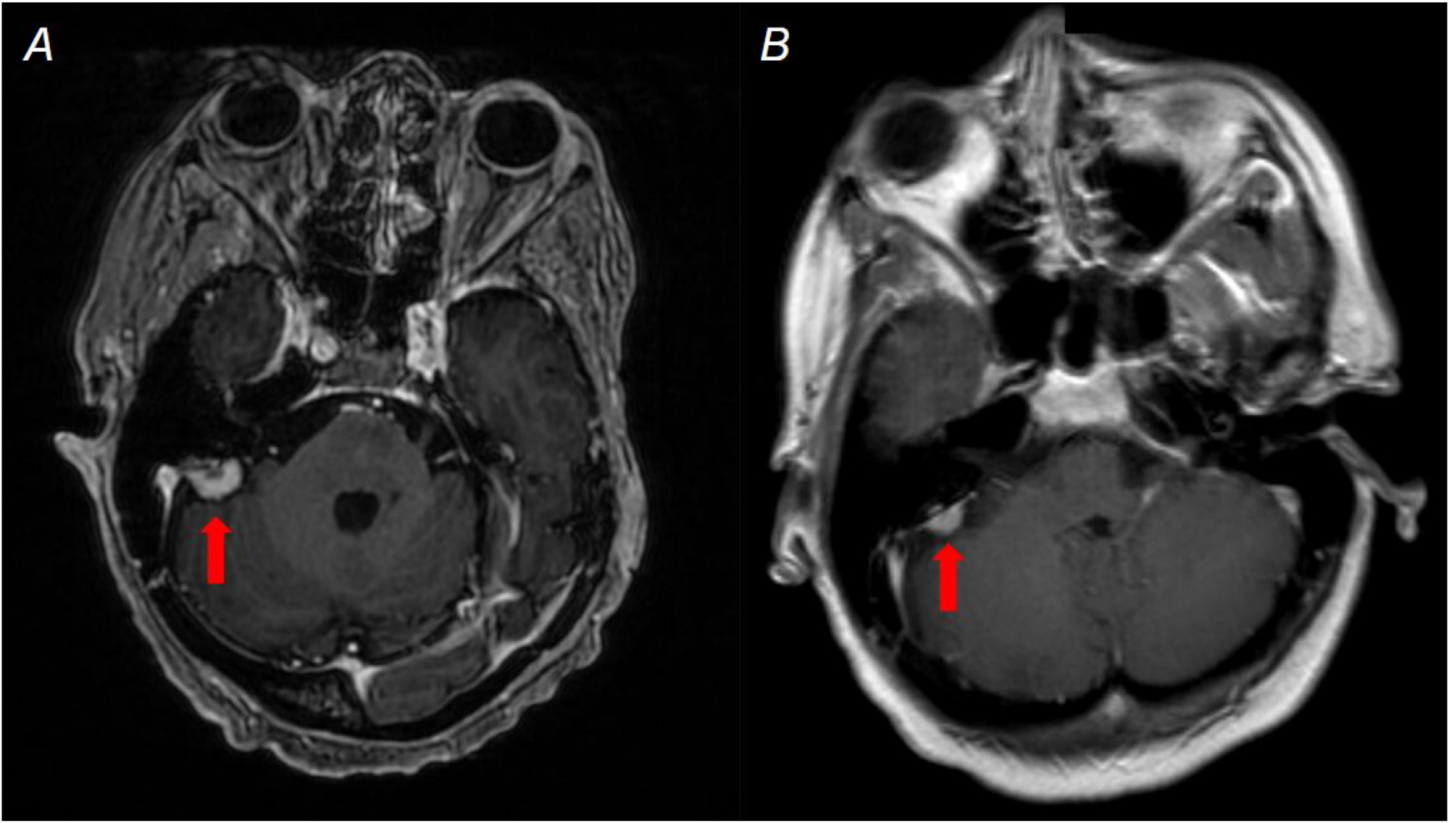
Brain MRI performed with and without contrast in 2025 shows a right petrous mass attached to the dura (red arrows). The mass demonstrates enhancement on axial postcontrast T1-weighted sequence and a dural tail, consistent with a meningioma (A). This was initially diagnosed in 2013 (B) and has been slowly growing. This was felt to reflect a radiation-induced meningioma given her history of childhood cranial radiotherapy.
